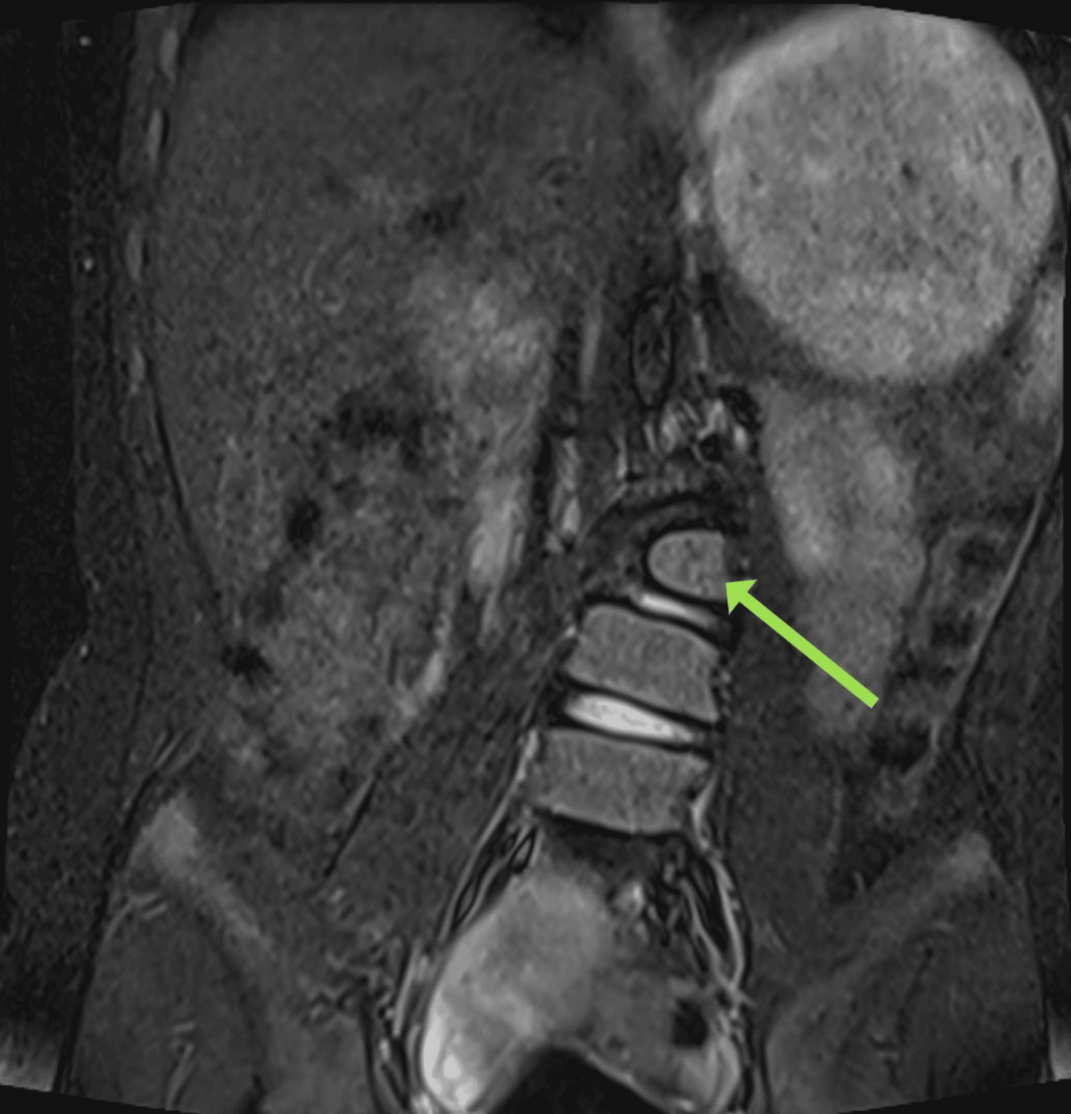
MRI image The arrow shows the L2 hemivertebra in the coronal section with levoscoliosis (Cobb's angle of about 25%).
